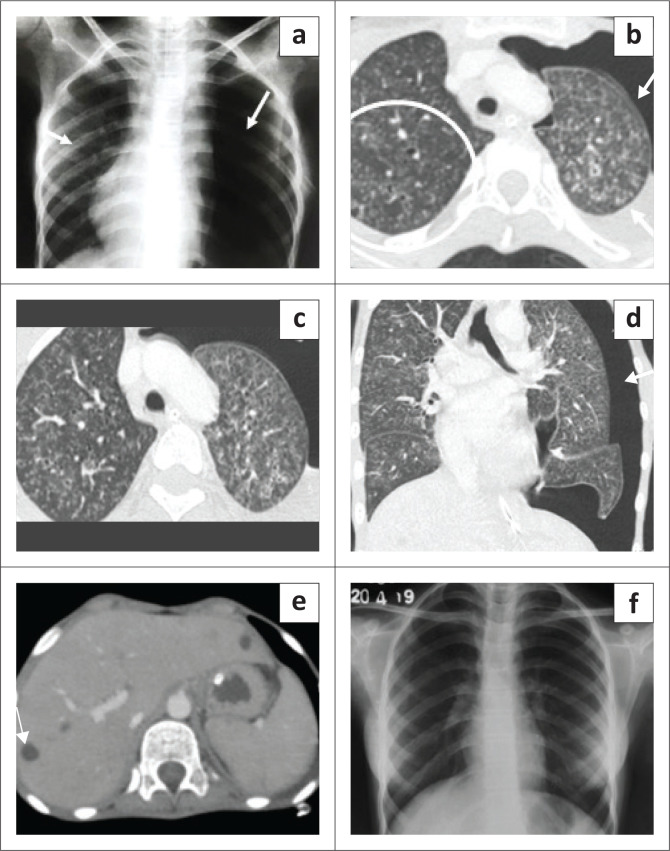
A 13-year-old lean, underweight female with disseminated tuberculosis. Chest radiography (CXR): (a) shows a left pneumothorax (long arrow) with the infiltrates in the right mid zone (short arrow). Contrast-enhanced CT chest axial (b, c) and coronal (d) images reveal a left hydropneumothorax (white arrow) with underlying collapse, multiple bilateral centrilobular nodules showing tree-in-bud opacities and a few tiny lung cysts (circle), better seen on the zoomed-up image (c). Axial section of the abdomen (e) reveals a few liver granulomas (arrow). (f) Follow-up CXR performed after 5 months appeared normal.
